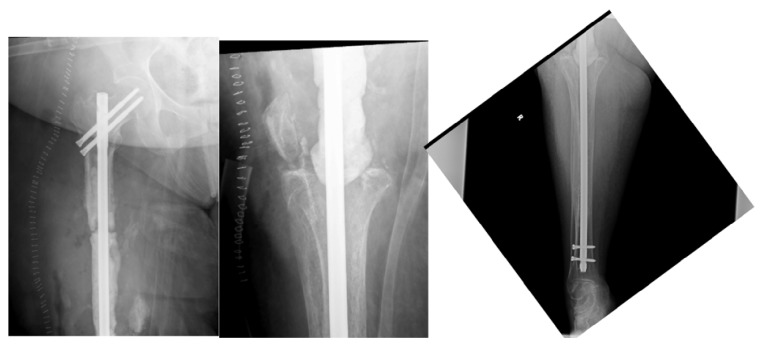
Postoperative radiographs showing long IM nail and surrounding bone cement.
